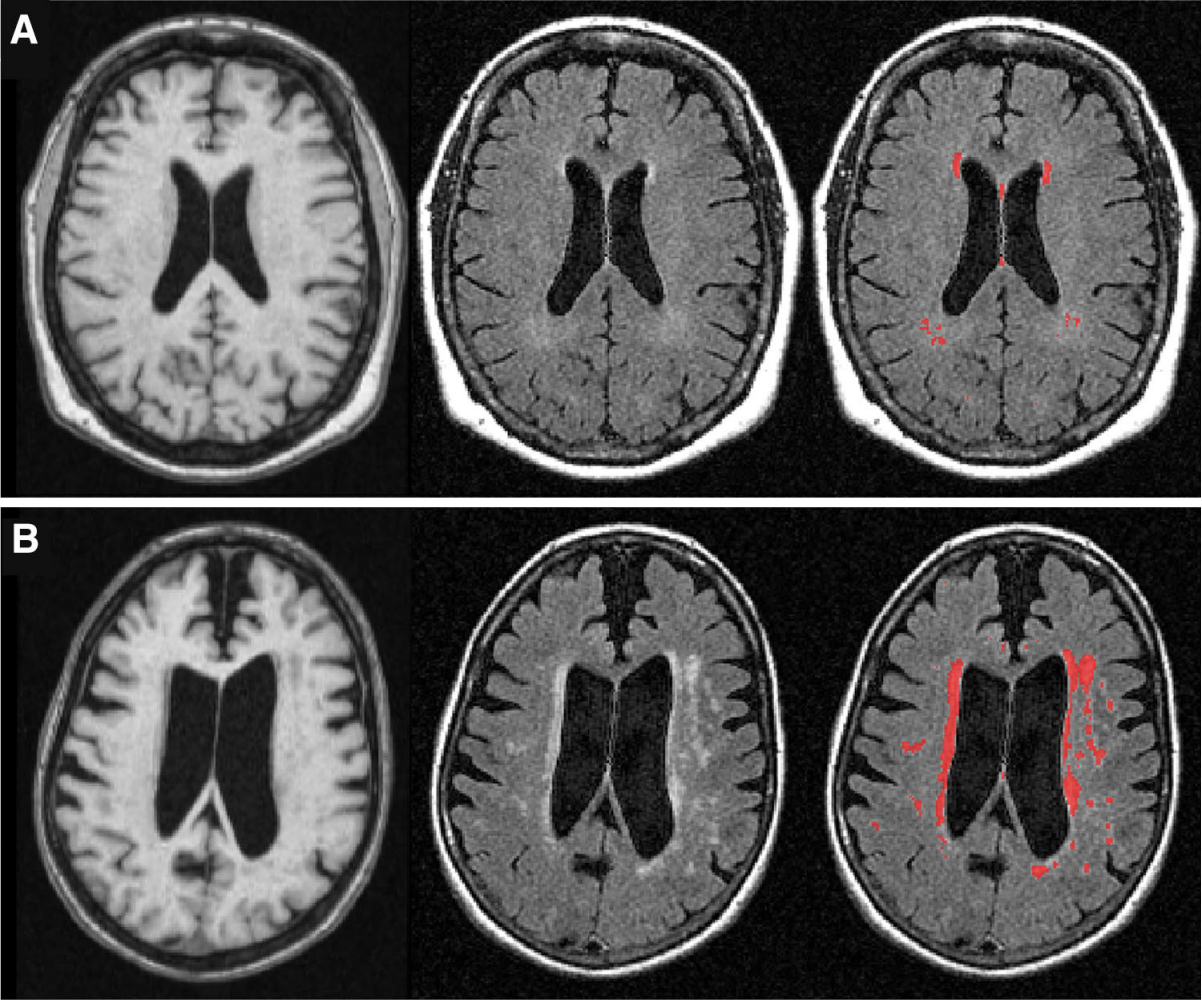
Brain MRI images from two individuals, one with small (a), and one with large (b) total WMH volume. *Top row* (a) shows one individual with small (25 %) total WMH volume, and *bottom row* (b) shows another individual with large (75 %) total WMH volume. The *left column* shows averaged *T*
